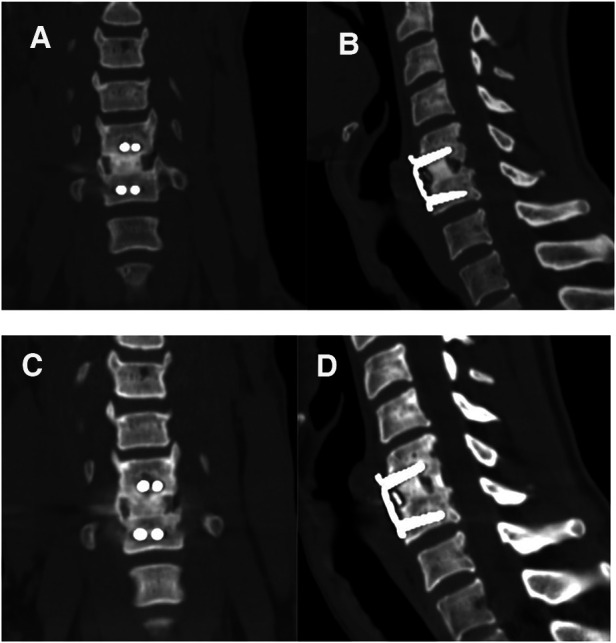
Coronal and sagittal computed tomography scans taken 12 months (A,B) and 36 months (C,D) after surgery showing satisfactory fusions.
